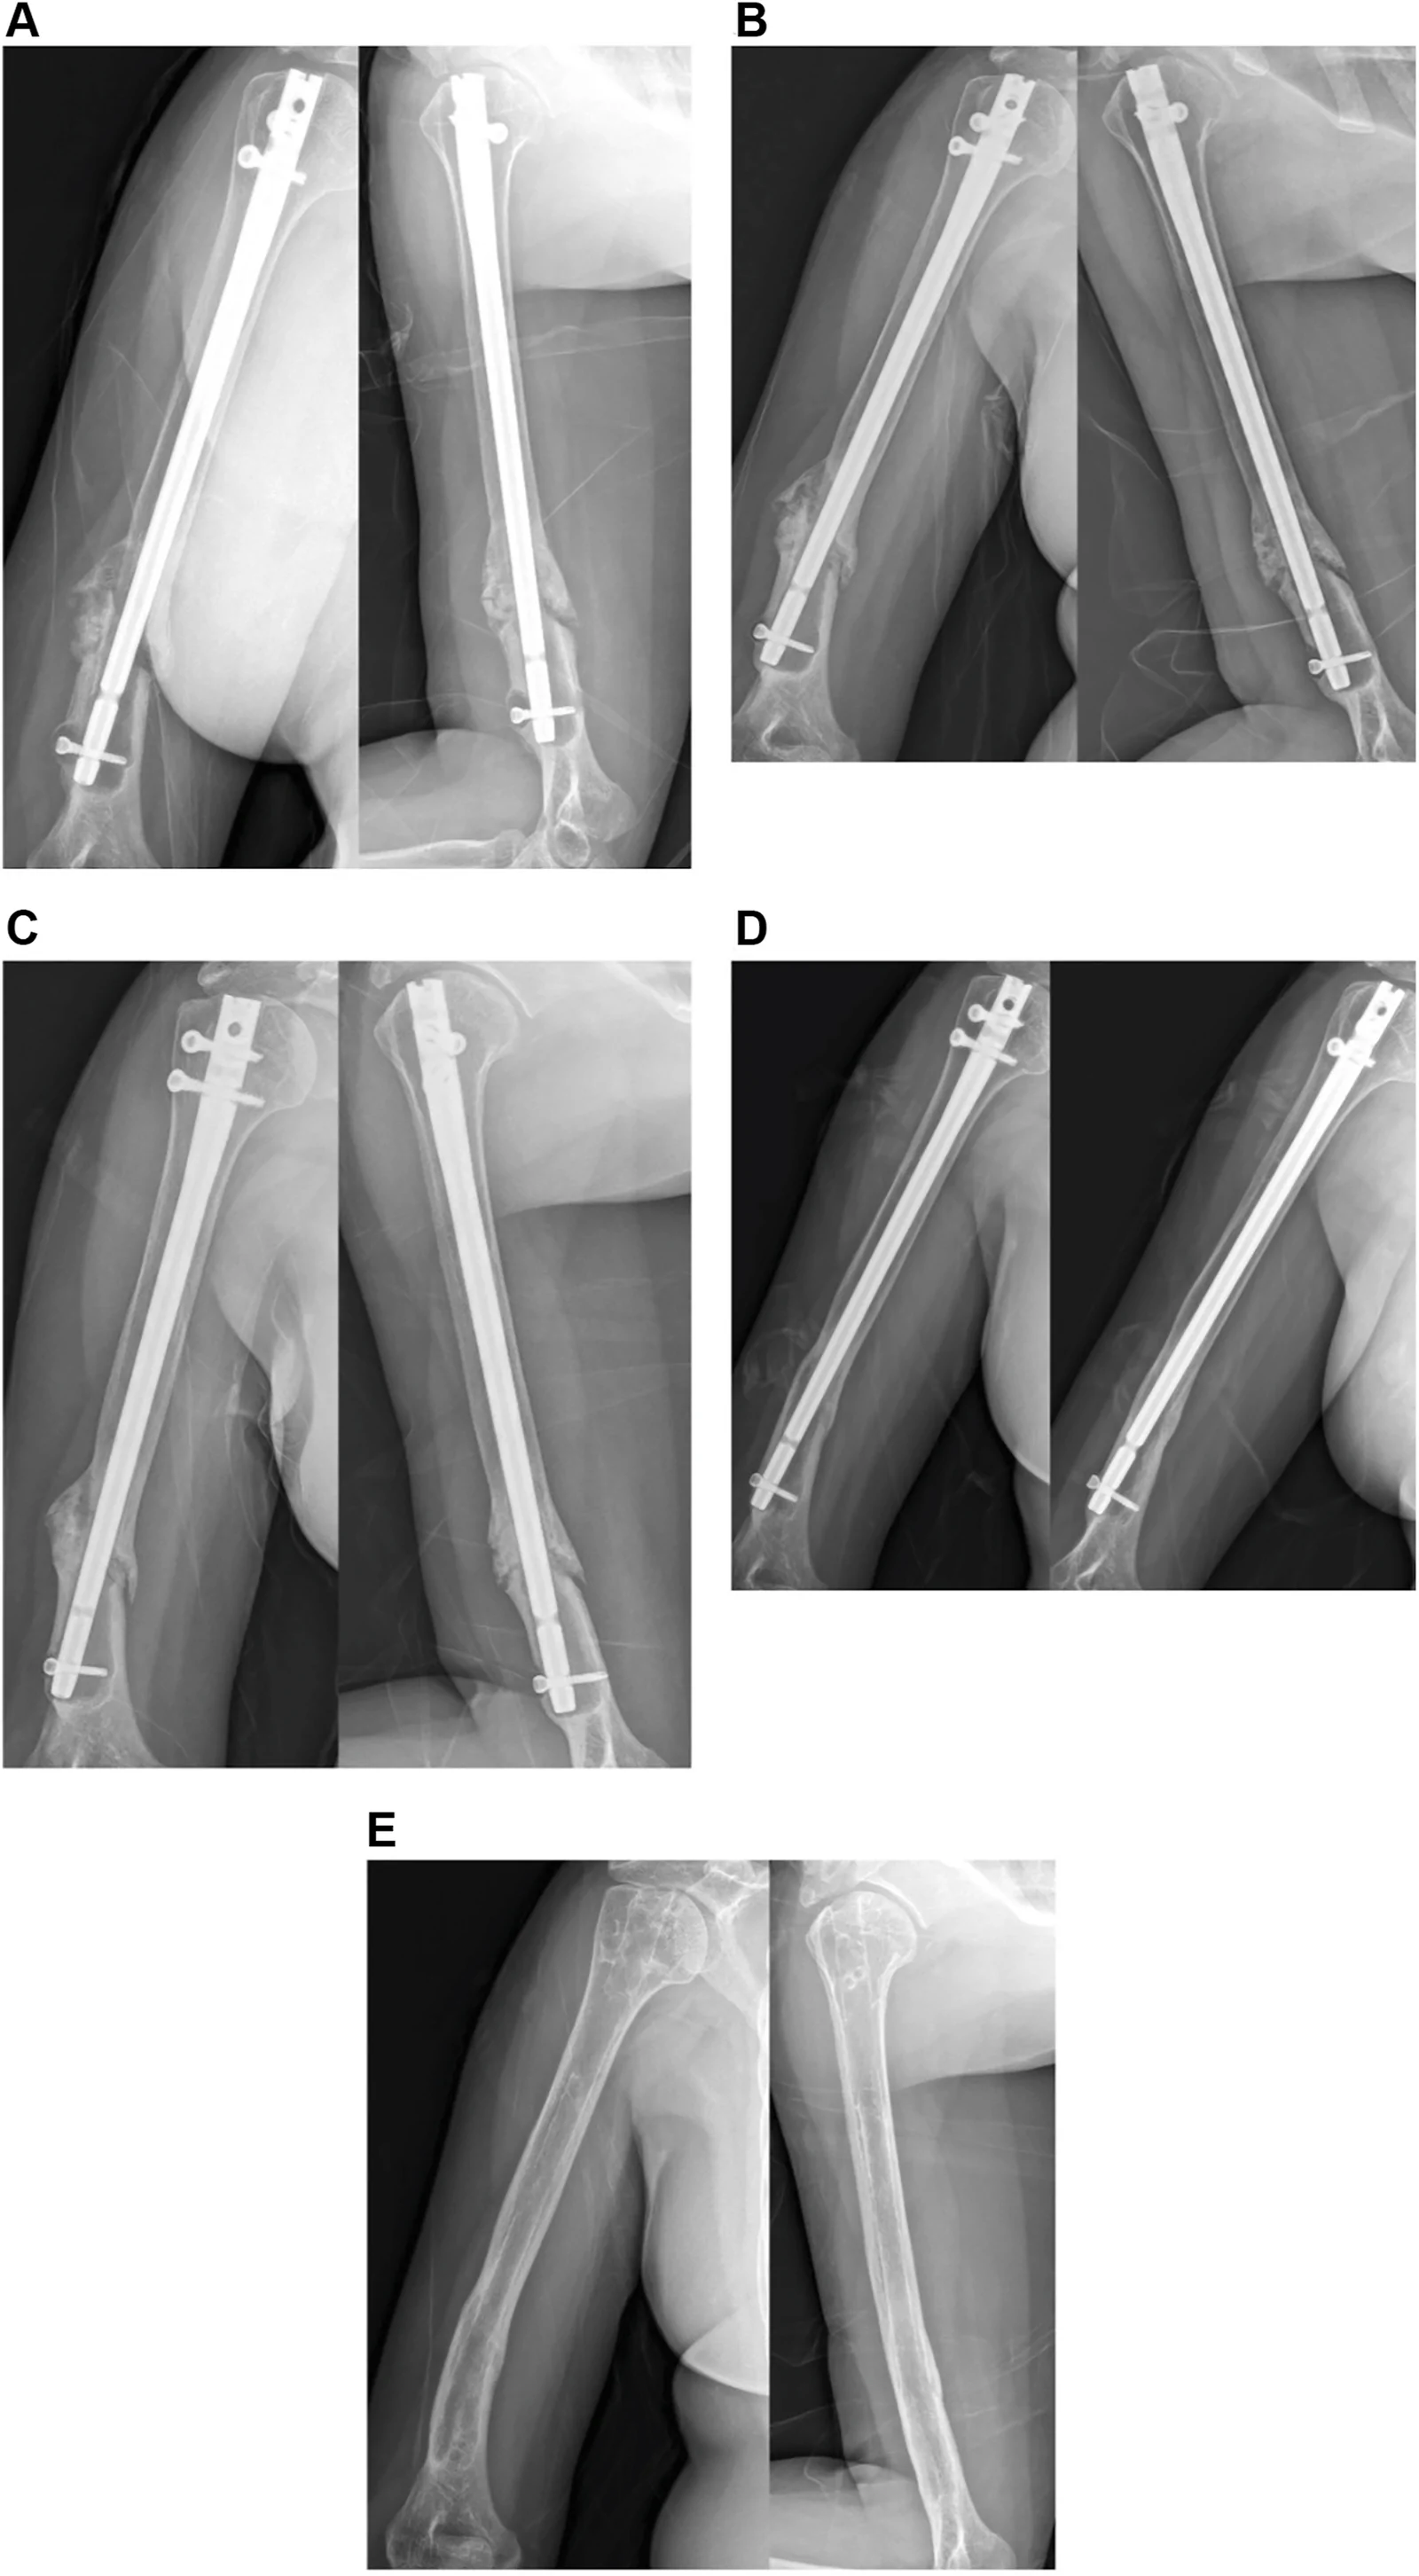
Serial radiographs of Case 1, a 49-year-old woman with late-stage non-union and intramedullary nail loosening after fixation for a right humeral shaft fracture. (A) Persistent non-union with implant loosening at 12 months post-operatively. (B and C) Progressive callus formation at 2 and 8 months after extracorporeal shock wave therapy (ESWT). (D) Further consolidation at 24 months after ESWT. (E) Radiograph after implant removal showing maintained alignment and union.
